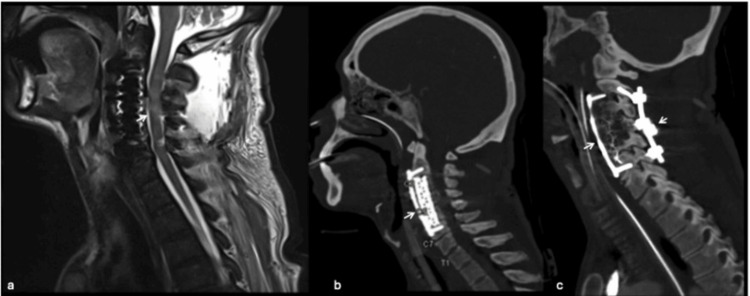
Postoperative imaging of a cervical chordoma following circumferential resection and reconstruction. (a) Sagittal T2-weighted MRI demonstrating adequate spinal cord decompression after tumor removal (arrow). (b) Sagittal CT scan showing anterior corpectomy with titanium mesh cage and anterior plate fixation extending from C2 to C7 (arrow). (c) Sagittal CT scan depicting posterior instrumentation with pedicle and lateral mass screws, bilateral rods, and cross-link, providing circumferential stabilization of the cervical spine (arrows).
